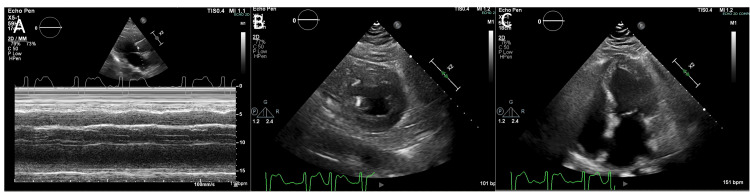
Echocardiogram depicting severely reduced left ventricular function with global hypokinesis A) Vertical long-axis view, B) horizontal short-axis view, and C) four-chamber view
